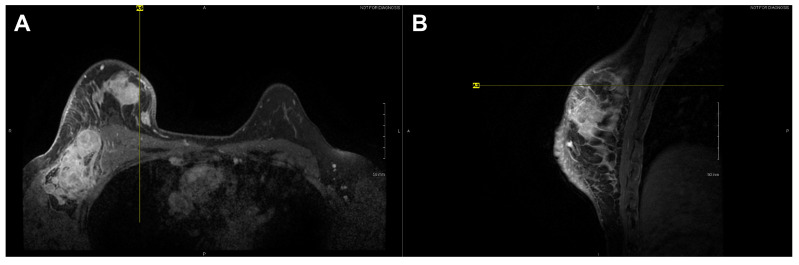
Axial (A) and sagittal (B) T1 contrast enhanced MR images of Subject 10’s malignant lesion. Linear enhancing vessels on sagittal images appear as enhancing round or oval foci on axial cross-sections.
